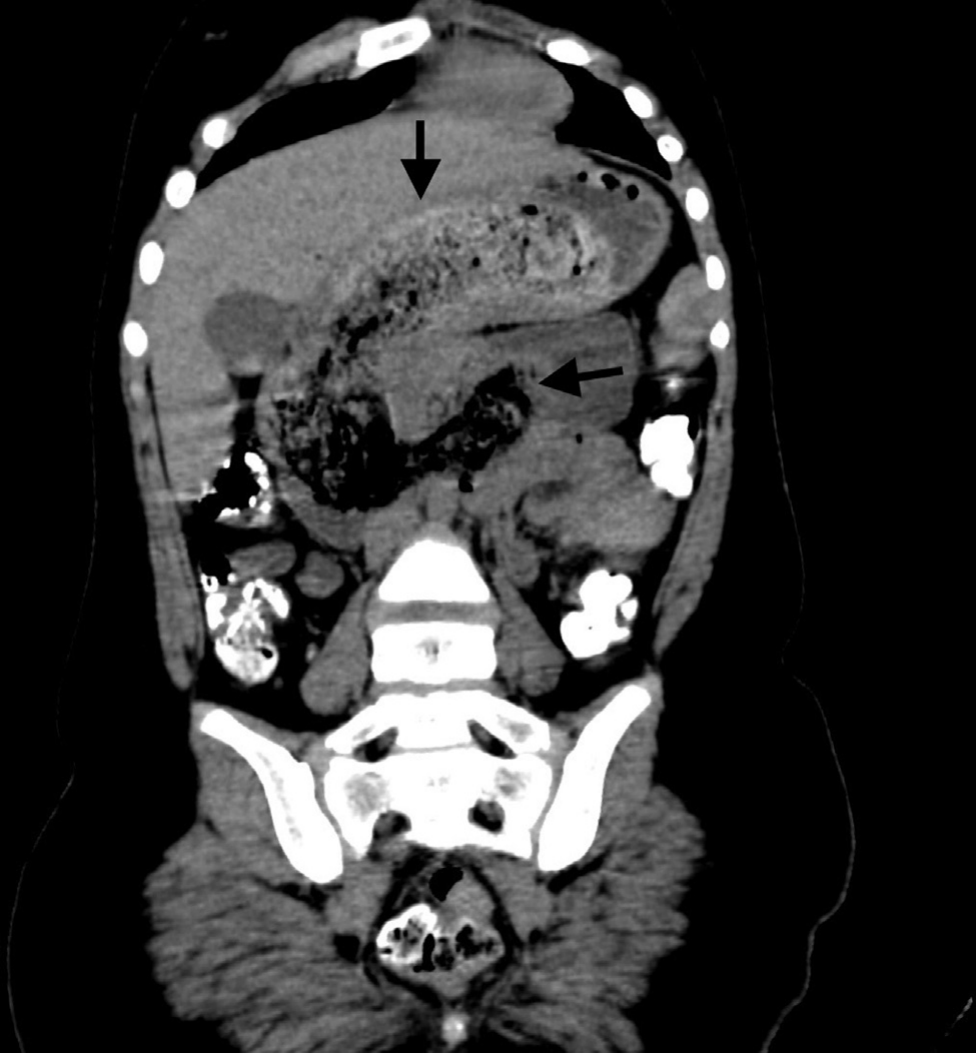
Coronal multiplanar reformation of oral and contrast-enhanced computed tomographic of abdomen demonstrating the trichobezoar (black arrows) outlined by oral contrast media and contents in the stomach, duodenum, and proximal jejunum.
